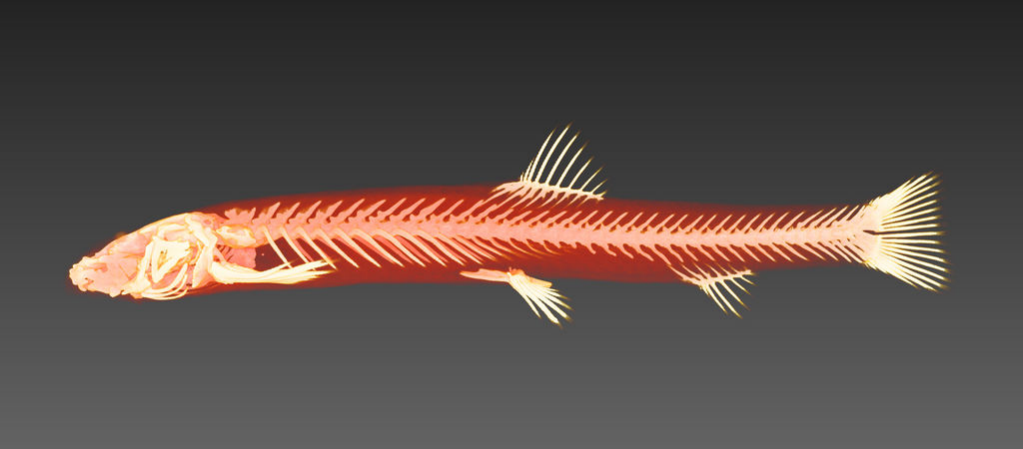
CT scanned data of Barbatula
